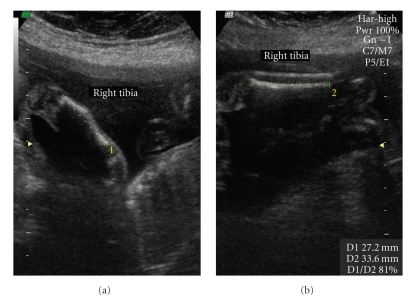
Ultrasound images showing absence of right fibula, bowing of right tibia (a), and tibial discrepancy (right tibia: 27.2 mm and left tibia: 33.6 mm) at 23 weeks gestation (b).
